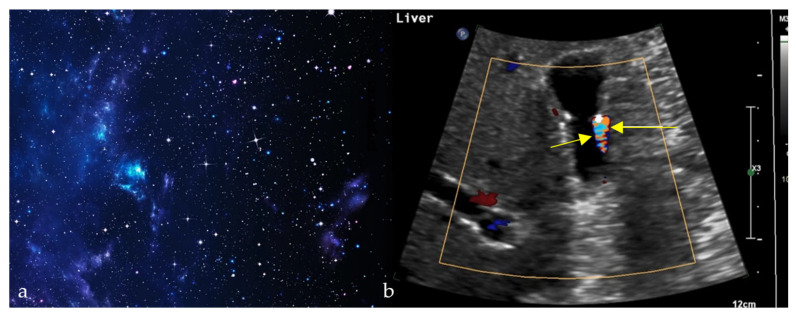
Alternating colors on Doppler signal behind a reflective object (such as a calculus) (yellow arrows) (b), giving the appearance of turbulent blood flow and resembling a twinkling sky (a).
